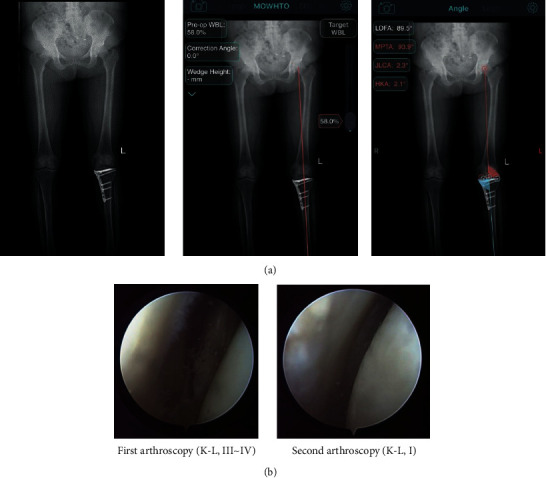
Full-length double-limb weight-bearing X-rays were taken for the assessment of the postoperative mFTA and mMPTA in the prementioned case, which were totally consistent with the target angles (a). The second arthroscopic look showed the cartilage degeneration recovered 18 months after surgery (b).
